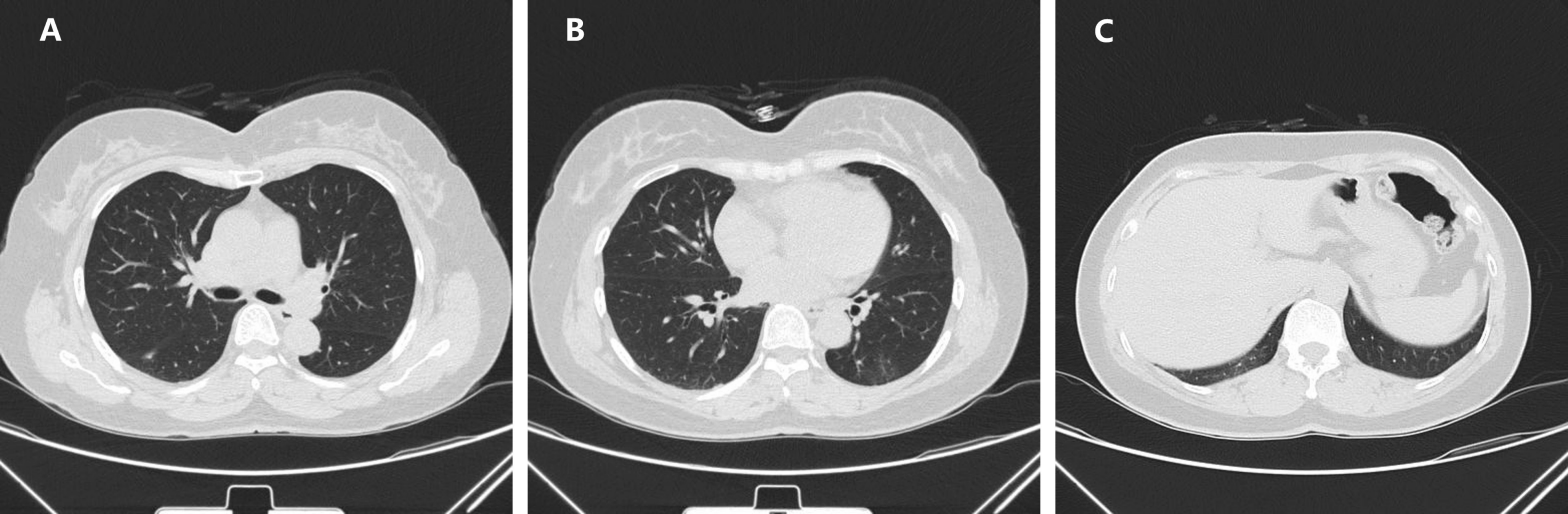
Follow‐up chest CT on May 20, 2025, showing resolution of the cavities in both lungs.
